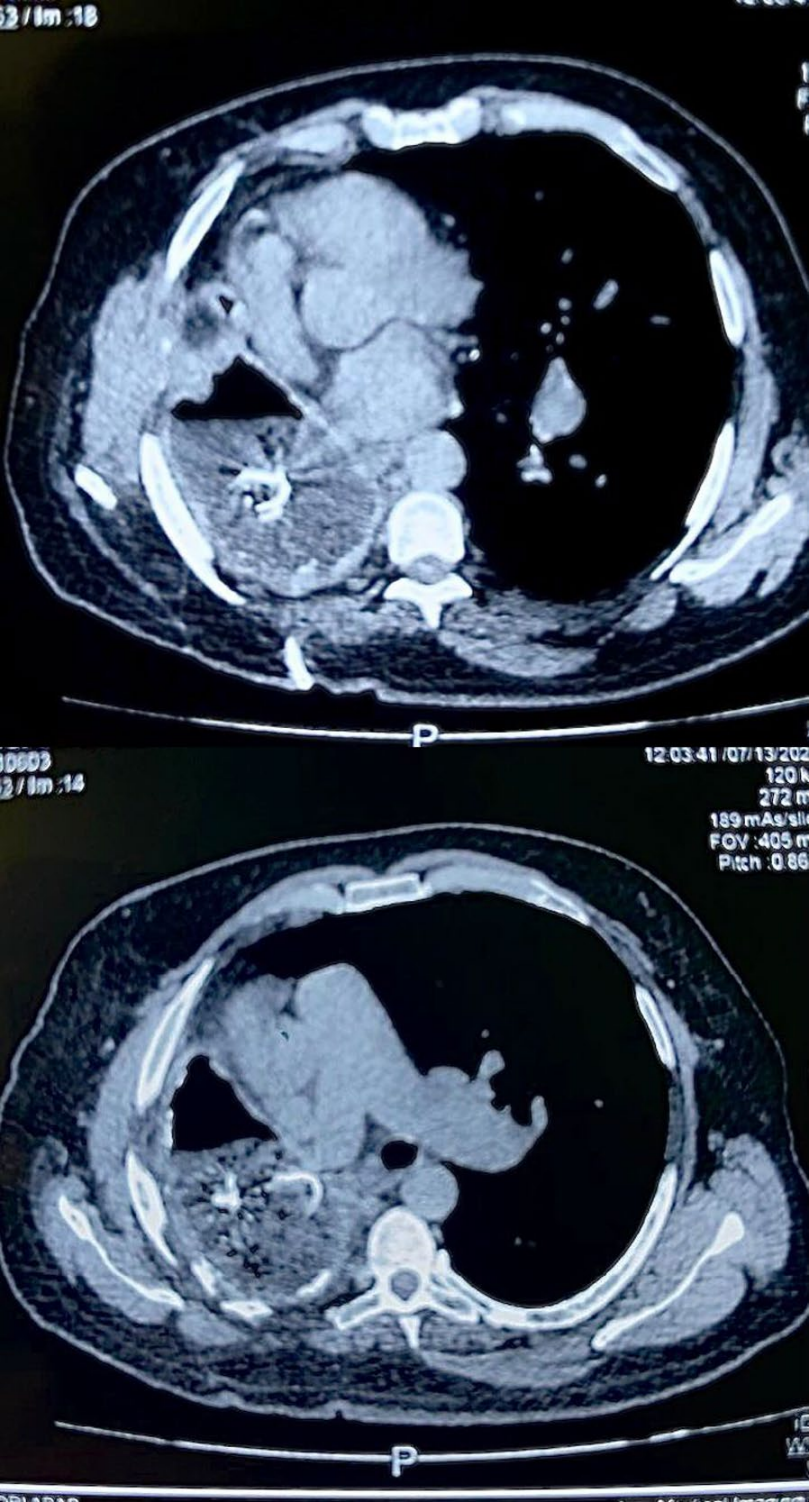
Axial thoracic CT scan showing gossypiboma in occupying right hemithoracic cavity
